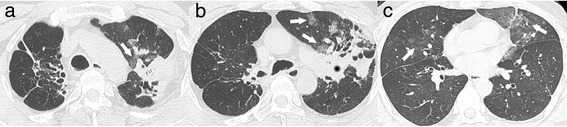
A 53-year-old man with hemoptysis and old inflammatory lesions. a–c Continuous high-resolution computed tomography scans showing old inflammatory lesions with traction bronchiectases and cicatricial volume loss in both the upper lobes and combined peribronchial consolidations (*star* in b) in the apicoposterior segment of the left upper lobe (LUL). Multifocal ground glass attenuations (GGAs, *arrows* in a–c) are seen in the LUL and right middle lobe (RML). The most prominent GGA site is the lingular segment of the LUL (*arrows* in c)
